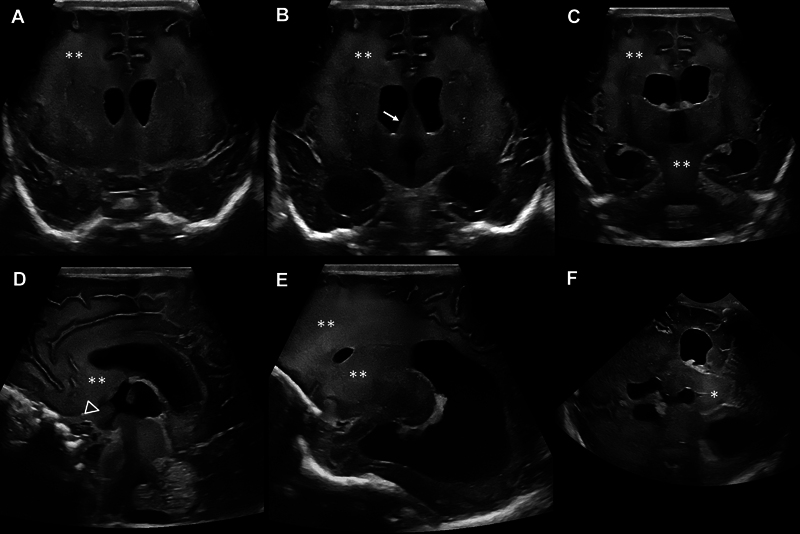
Cranial ultrasound on DOL 32. Progressive increase of white matter echogenicity with very fine, homogeneous echo texture (two asterisks) with frontal predominance (
